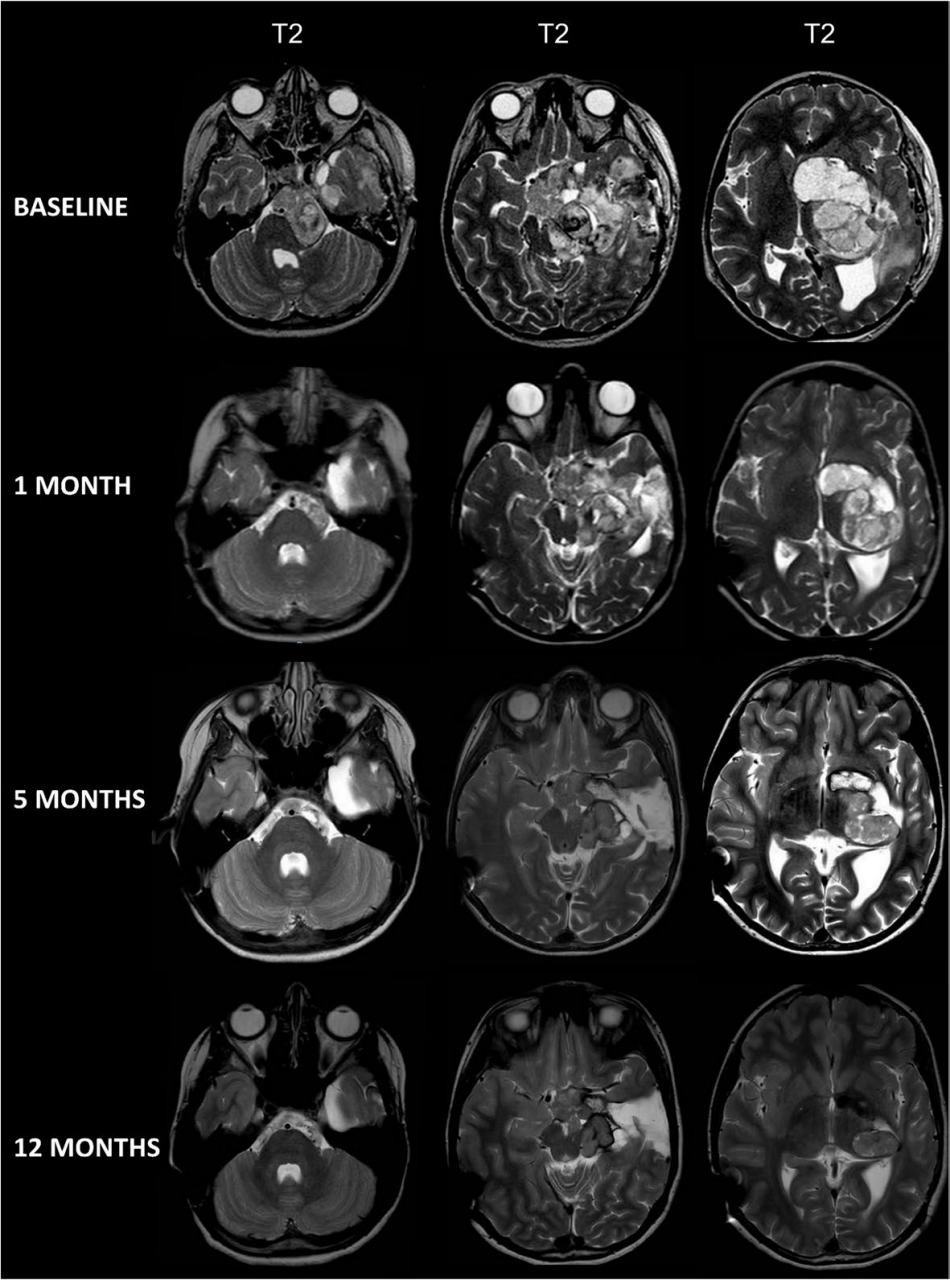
Brain MRI of the 12-year-old girl with ganglioglioma. T2-weighted axial sequences at baseline (after biopsy) show a heterogeneous mass with epicenter in the hypothalamic-chiasmatic region, prominent involvement of the left basal ganglia and thalamus, and caudal exophytic extension with midbrain compression. Follow-up MRI at 1 month from starting vemurafenib shows quick and evident shrinkage (-45%, according to RANO criteria) with prominent regression of the left prepontine component. Mass reduction continues at 5 months (-70%) and is stable at 12 months (-76%)
